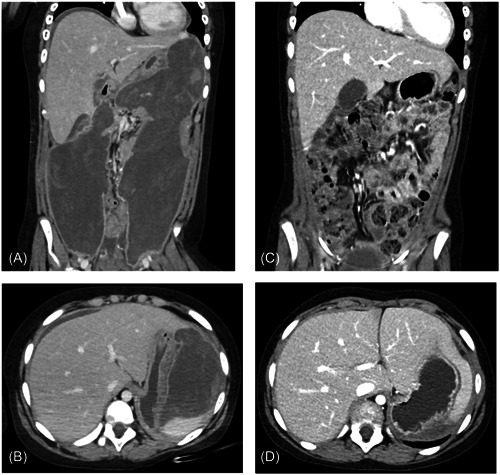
(A, B) Computed tomography (CT) abdomen and pelvis obtained upon admission, coronal and axial views, respectively. Evidence of severe pancreatitis with walled‐off necrosis in the bilateral paracolic gutters extending to the mid‐pelvis. (C,D) CT abdomen and pelvis obtained after discharge (53 days after the CT depicted in Images [A, B]) from the hospital. Significant interval improvement in both pancreatitis and in the size of the walled‐off collection is shown.
